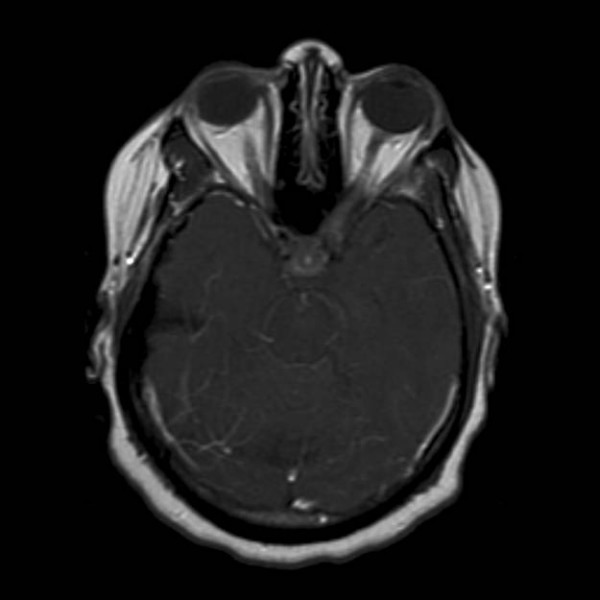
MRI Brain with contrast demonstrating improvement 2 months after initiation of thalidomide.
